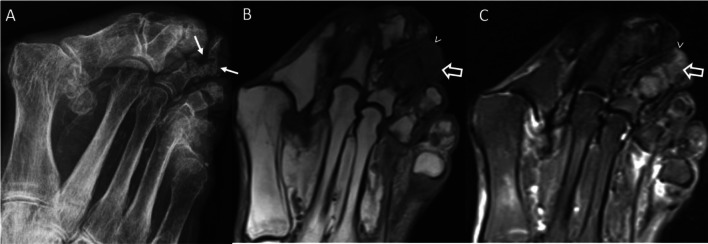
Osteomyelitis of the third toe in a SLE patient. Conventional radiography A shows diffuse bone destruction of middle and distal phalanges of the third toe (arrows). Magnetic resonance imaging shows diffuse low T1-weighted signal (B; open arrow) and increased STIR signal (C; open arrow) within the medullary cavity of the intermediate and terminal phalanges of the third toe, which is associated with marked soft tissue swelling (arrowheads)
